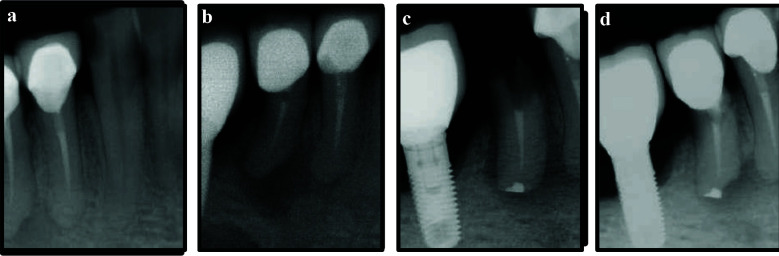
a, Periapical radiograph of tooth 45 immediate post-endodontic treatment; b, Two years post-endodontic treatment; c, Immediate post intentional replantation; d, Five months follow-up.
